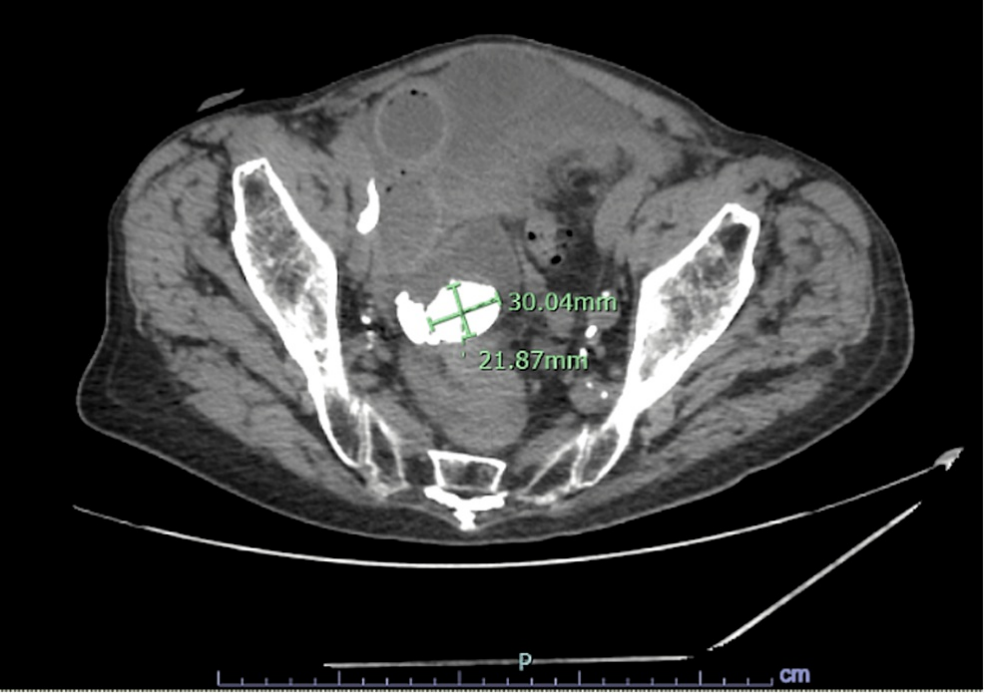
Abdominal and pelvic CT scan showing a 3 cm stone located in a distended loop of the small bowel in the pelvis CT: computed tomography
